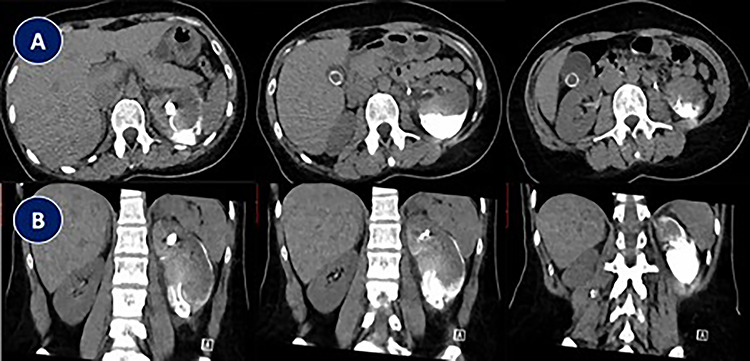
Abdominal CT scan showing complete misplacement of DJ stent into subcapsular renal space. (A) CT scan image (Axial). (B) CT scan image (Coronal).
